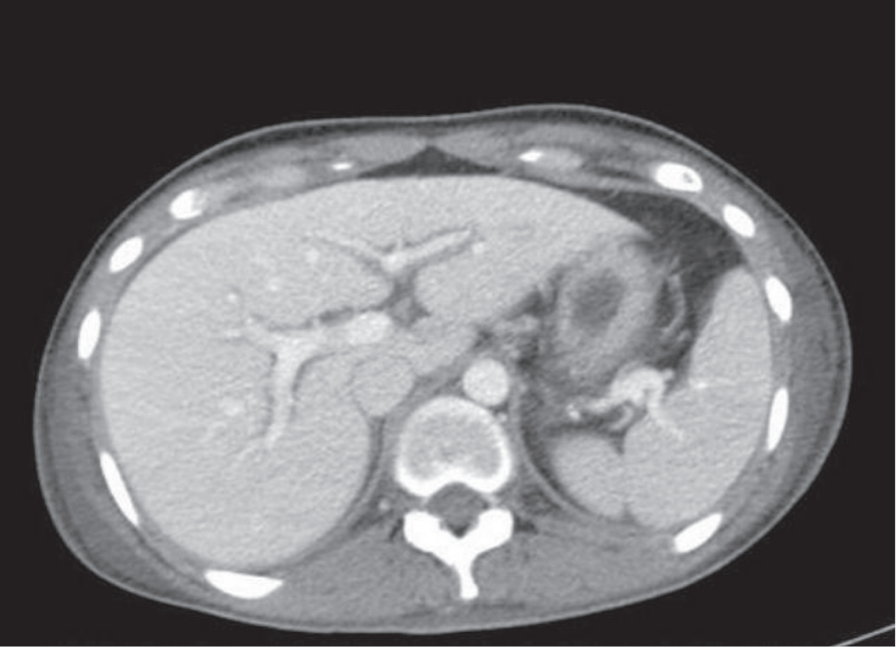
Contrast-enhanced computed tomography scan showing axial section of the patient’s upper abdomen. Note the peri-portal hypodensity at the level of the hilum, which is confined to the main portal veins and the first-order branches, consistent with peri-portal lymphedema.
